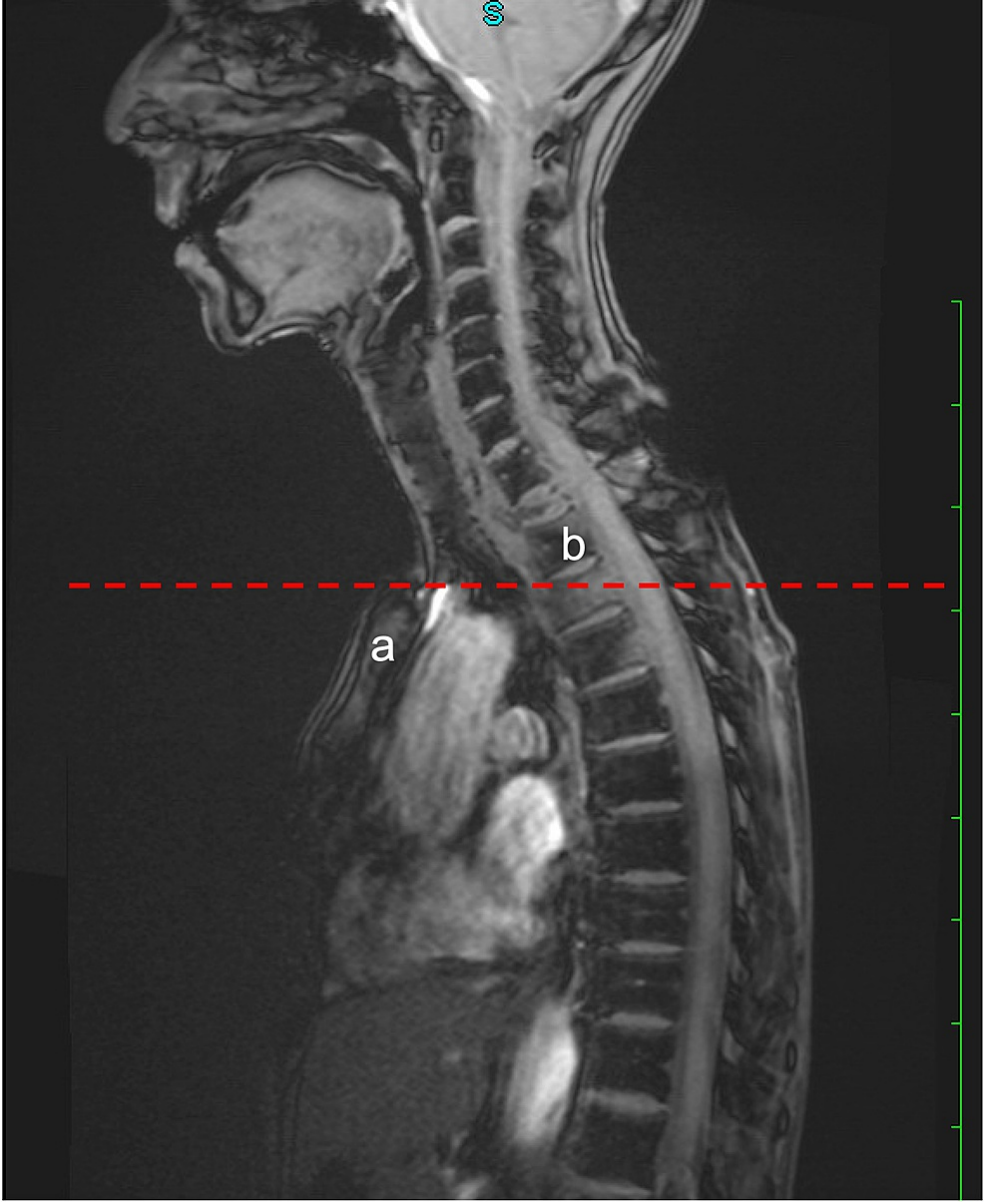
Sagittal MRI of the thoracic spine A tangential line is drawn superior to the sternal notch (a) and inferior to the level of the T3 vertebra (b).
